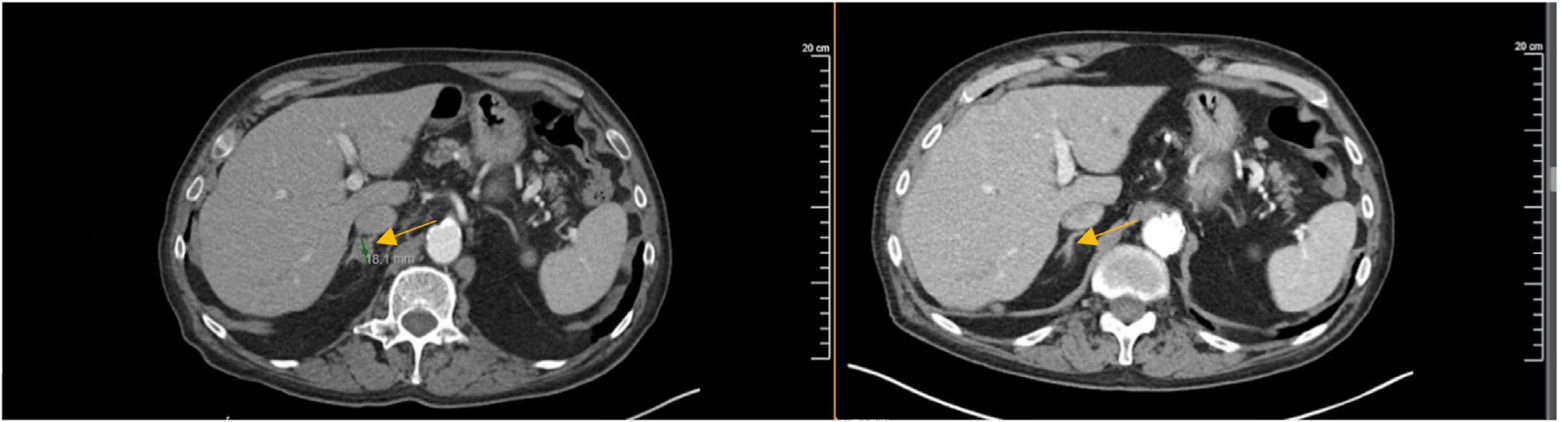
CT scan of an adrenal nodule before and after PARPi treatment. CT scan (*left* scan) taken before PARPi therapy course showing a 1.8 cm adrenal nodule, which demonstrated reduction following treatment (*right* scan). *Yellow arrow* pinpoints the adrenal nodule before and after therapy.
